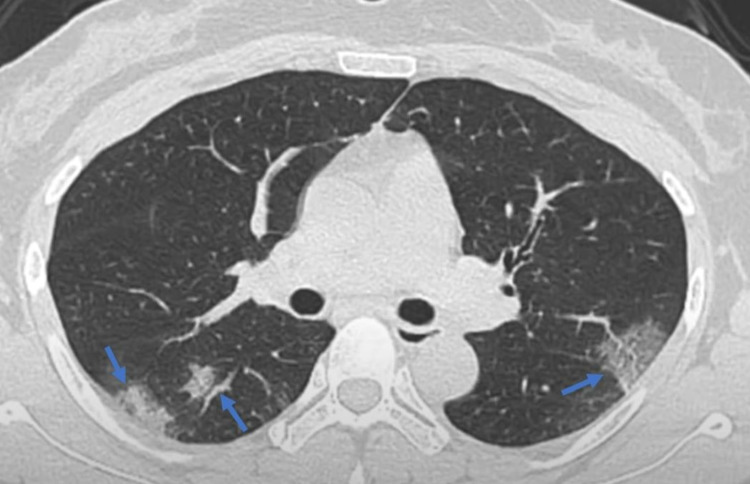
CT chest showing patchy opacities in both lungs
